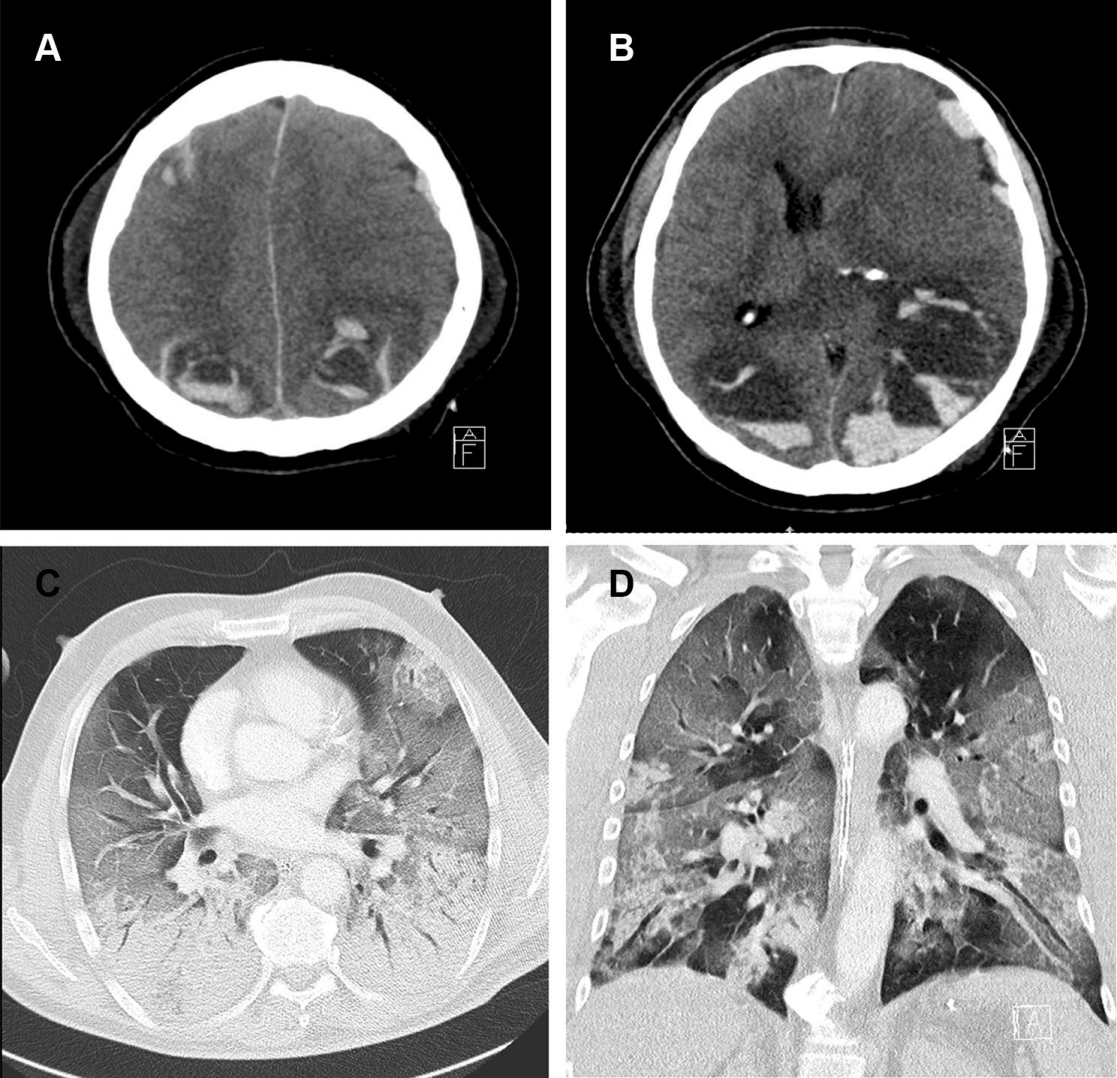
Imaging (CT) of the patient in the case report. a Bilateral hemorrhage in the parietooccipital parenchyma featuring fluid–fluid levels and left-hemispheric subdural hematoma. Considerable mass effect resulting in significant midline shift to the right as well as transtentorial and subfalcine herniation. b Right-sided subarachnoidal hemorrhage adjacent to small intraparenchymal bleeding. c, d Extensive ground glass opacities predominantly in the periphery of both lungs and dorsal air space consolidation
